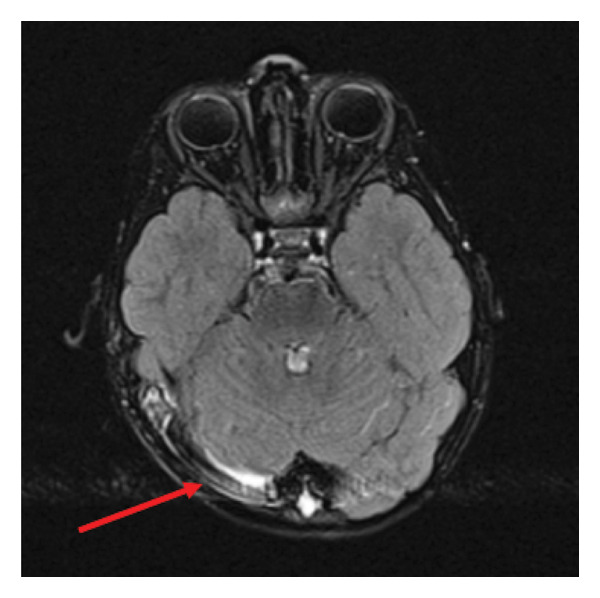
Brain MRI—lamellar collections along the sigmoid sinus (right‐sided).
